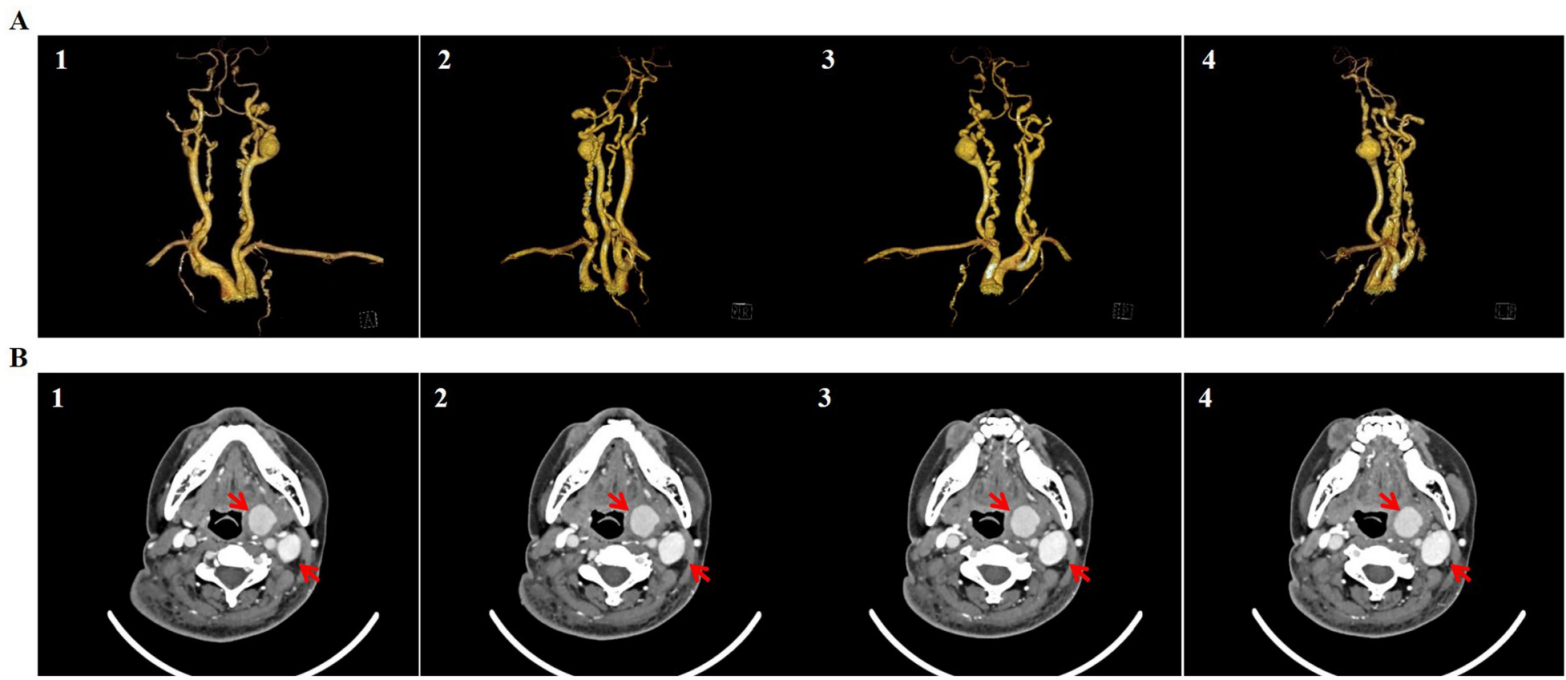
Fibromuscular dysplasia affected the intracranial, vertebral, carotid, and internal mammary arteries. A CT angiography revealed alternating areas of aneurysm and stenosis, described as a “string-of-beads” appearance in the intracranial, vertebral, carotid, and internal mammary arteries. The three-dimensional CT angiographic images are in the anterior (A1), right (A2), posterior (A3), and left (A4) views. The cross-sectional CT images indicate a hematoma on the left side of the laryngeal cavity and a large aneurysm in the left internal carotid artery (B) (indicated by arrows).
